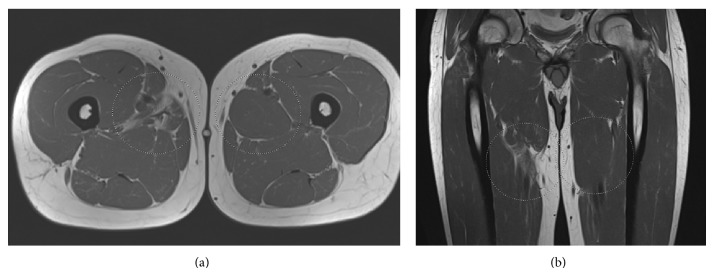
(a) T1-weighted transversal MRI image shows a minimal hyperintense area where the rupture in the adductor longus was seen on previous MRI images after the initial trauma. (b) T1-weighted coronal MRI image, additional to (a).
